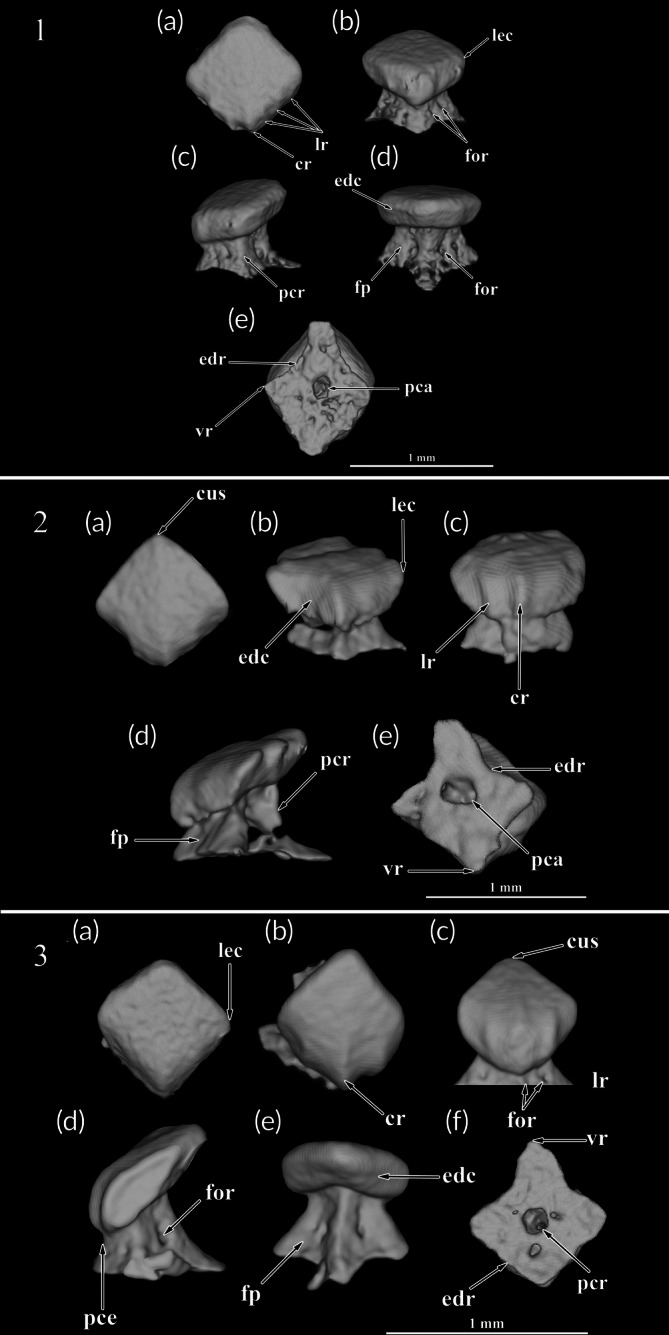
1 = Fin type 1 denticles (UFPB 14343 female, 1068 mm PCR). (1a) F1 dorsal view. (1b) F1 frontal view. (1c) F1 lateral view. (1d) F1 posterior view. (1e) F1 ventral view. In (1a, 1e) anterior to bottom; In (1b) anterior to front; In (1c) anterior to left; In (1d) anterior to back. 2 = Fin type 2 denticles (UFPB 14343 female, 1068 mm PCR). (2a) F2 dorsal view. (2b) F2 frontal view. (2c) F2 frontal view. (2d) F2 lateral view. (2e) F2 ventral view. In (2a, 2e) anterior to bottom; In (2b, 2c) anterior to front; In (2d) anterior to left. 3 = Fin type 3 denticles (UFPB 14343 female, 1068 mm PCR). (3a) F9 dorsal view. (3b) F5 dorsal view. (3c) F5 frontal view. (3d) F5 lateral view. (3e) F5 posterior view. (3f) F9 ventral view. In (3a, 3b, 3f) anterior to bottom; In (3c) anterior to front; In (3d) anterior to left; In (3e) anterior to back (micro CT‐scan).
